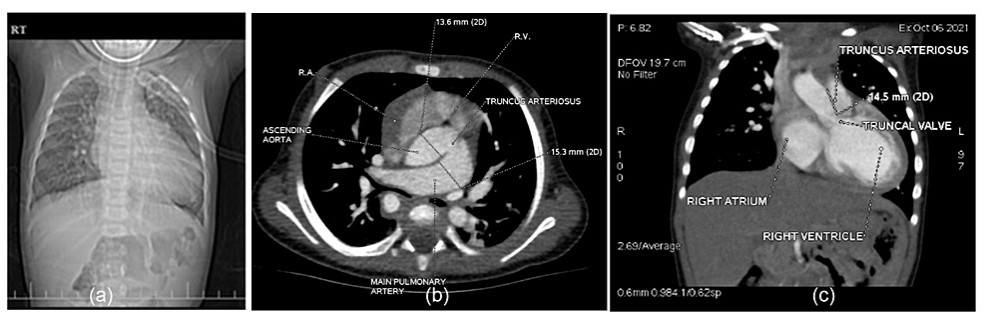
(a) Scannogram of chest AP: widened superior mediastinum with plethoric lung fields; (b) contrast-enhanced cardiac CT axial image: truncus arteriosus C & E type 1 and Van Praagh type A1; (c) contrast-enhanced cardiac CT coronal image: truncus arteriosus C & E type 1 and Van Praagh type A1 with common trunk having a length of 14.5 mm
